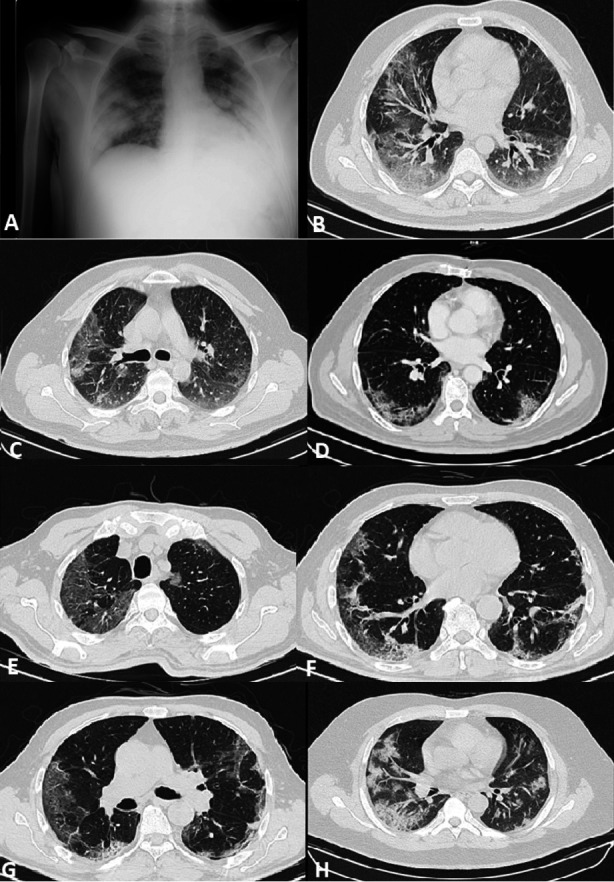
(A) Chest X-ray supine view of a 45 years male patient with severe dyspnea showing bilateral mid and lower zone airspace shadowing with peripheral predilection. (B) HRCT chest lung window axial images of same patient showing bilateral peripheral subpleural areas of ground glass haze with lower lobe predominance. (C,D) CT chest contrast axial image in lung window showing subpleural ground glass haze, reticulation and crazy paving in lower lobes in a 65 years male patients with fever, cough and sore throat. (E-G) HRCT chest lung window axial images of a 60 years male patient with fever, cough, dyspnea and body aches showing bilateral ground glass haze and crazy paving in peripheral subpleural location of both lungs. (H) HRCT chest lund window image of a 48 years male patient with fever, dyspnea and body ache showing bilateral peripheral subpleural consolidations with minimal ground glass haze in both lungs.
